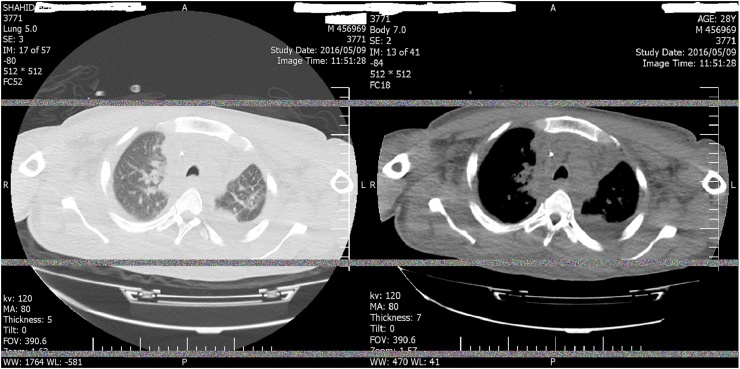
Axial lung and mediastinal window CT Scan, after 18 days show loculated empyema left hemi thorax with pericardial thickening (7 mm) after 18 day the first operation.
